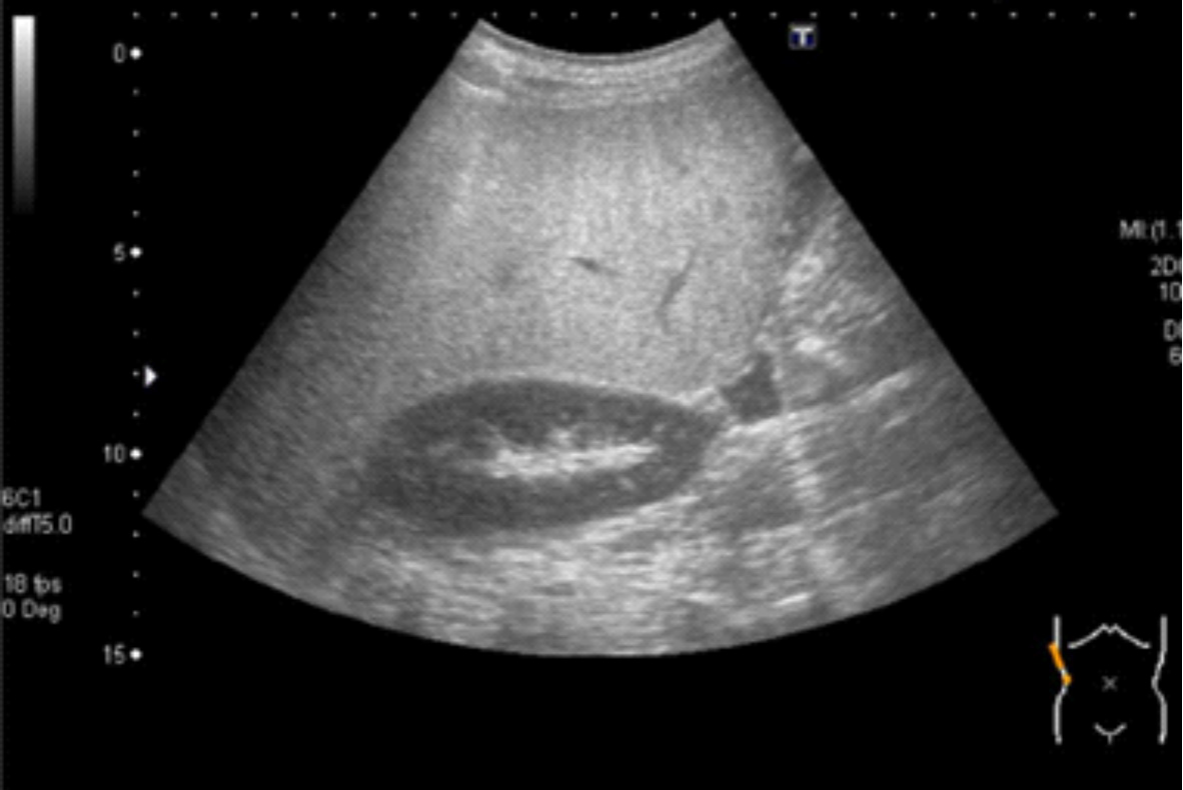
Abdominal ultrasound. Abdominal ultrasound revealed increased echogenicity in the liver compared with that in the kidney and spleen.
